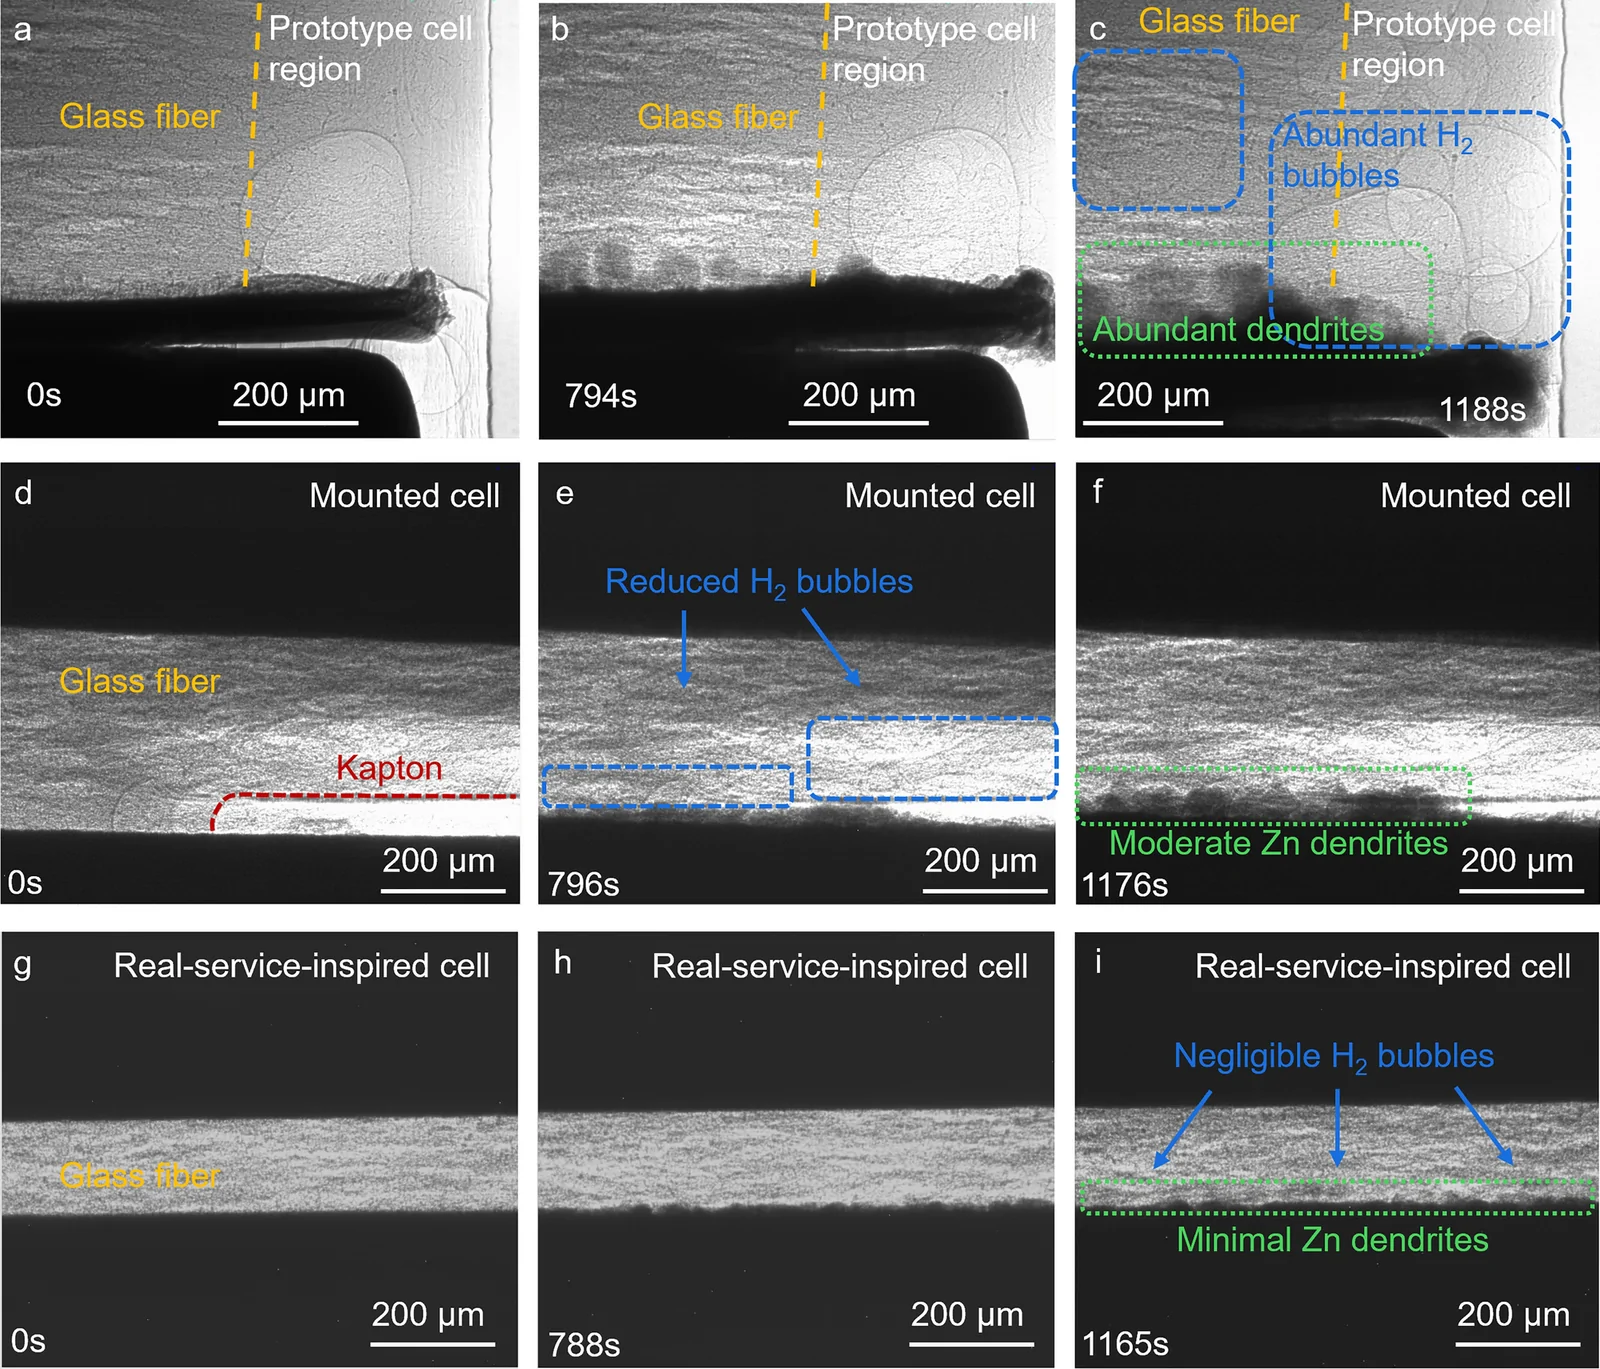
Operando* X-ray radiography images of electrode–electrolyte interface within Swagelok cells. The galvanostatic discharge current density is 15 mA cm^−2^. a-c Regions with and without (Prototype cell) glass fiber separators indicated by the orange dash lines. d-f Mounted cell. g-i Designed real-service cell
